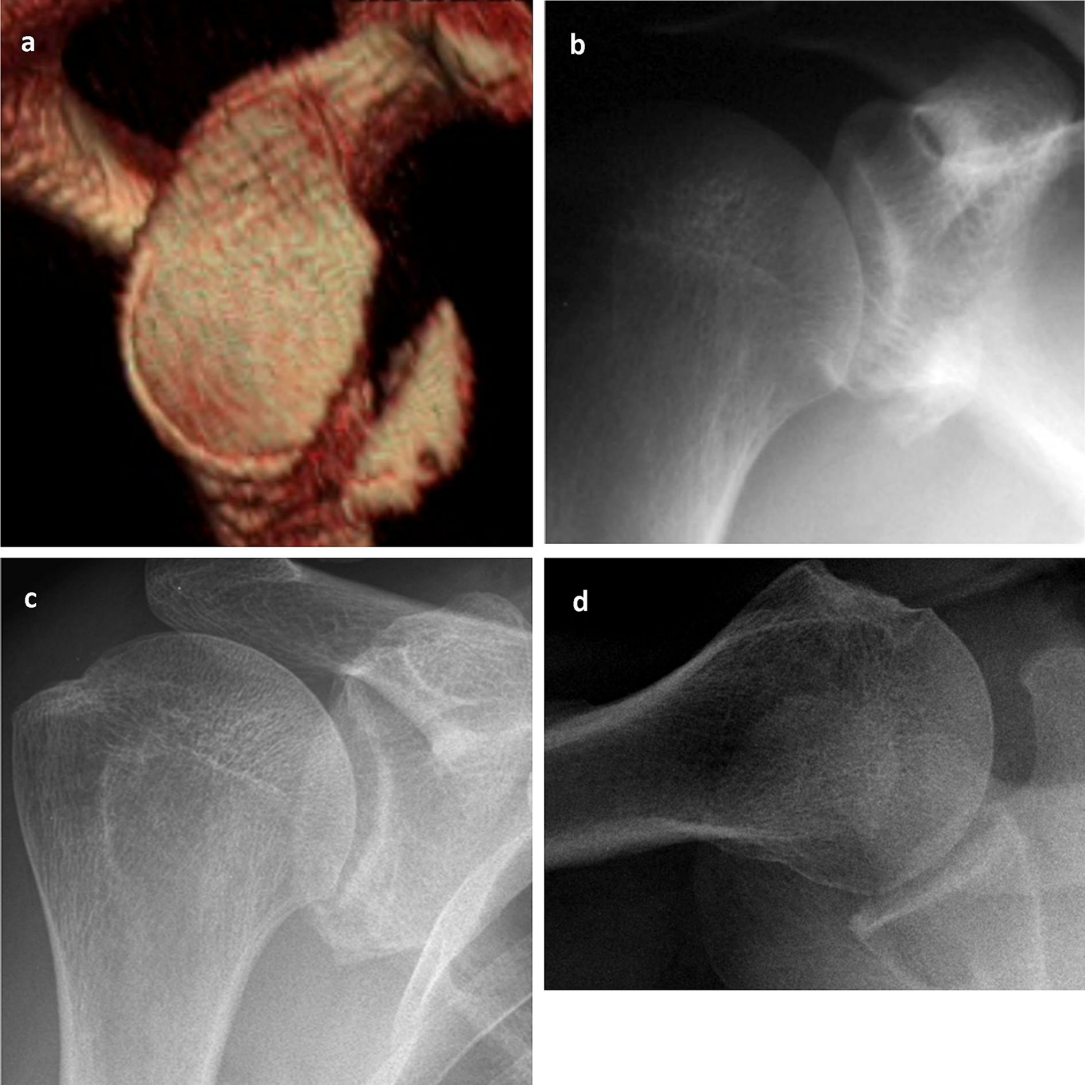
Non-operative treatment of an anterior GRF (a 3D-CT, enface view) in a 56-year-old patient after shoulder dislocation with a concentrically reduced humeral head (b A.P.-radiograph) without redislocation or signs of instability across the remaining course of 6 years and a healed fragment (c, d A.P. and axial-radiograph)
